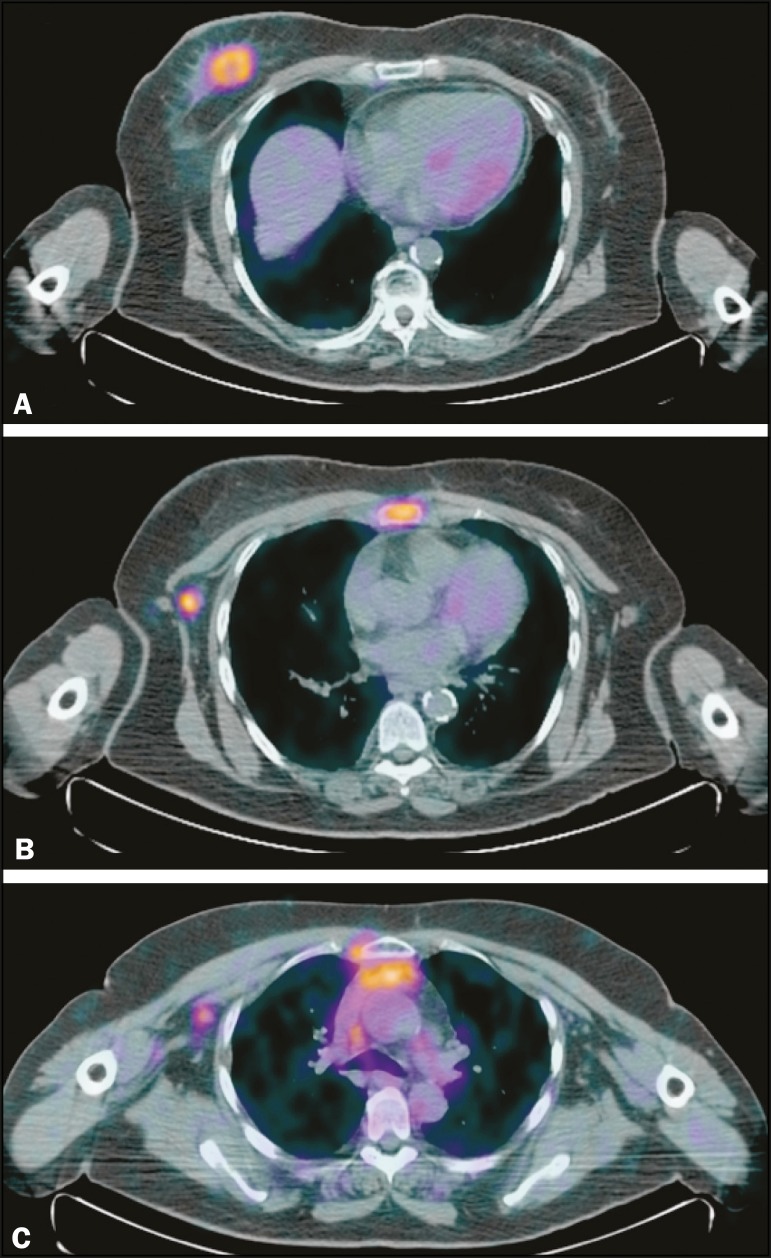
64-year-old woman with breast cancer. ^18^F-FDG PET/CT showed
